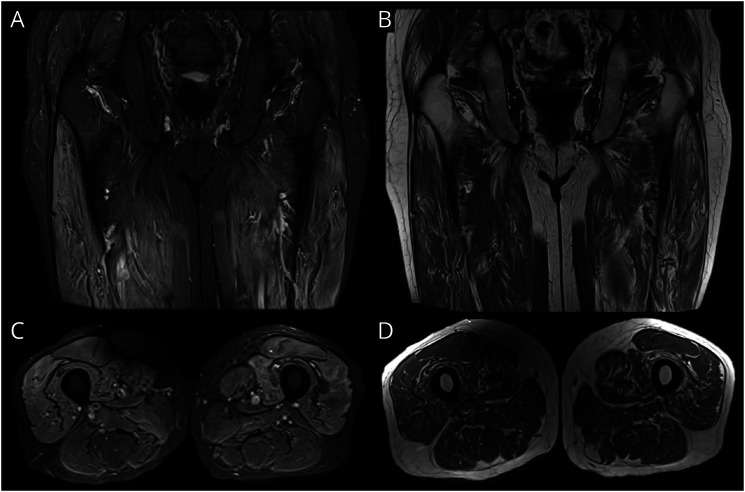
MR Imaging in a Patient With HTLV-1–Related Myositis Coronal and axial T2-weighted fat-saturated (A and C) and T1-weighted (B and D) images of a 70-year-old man with HTLV-1 and progressive myopathy over several years demonstrates edema and fatty infiltration into the anterior compartments of both thighs with relative sparing of the rectus femoris muscles.
